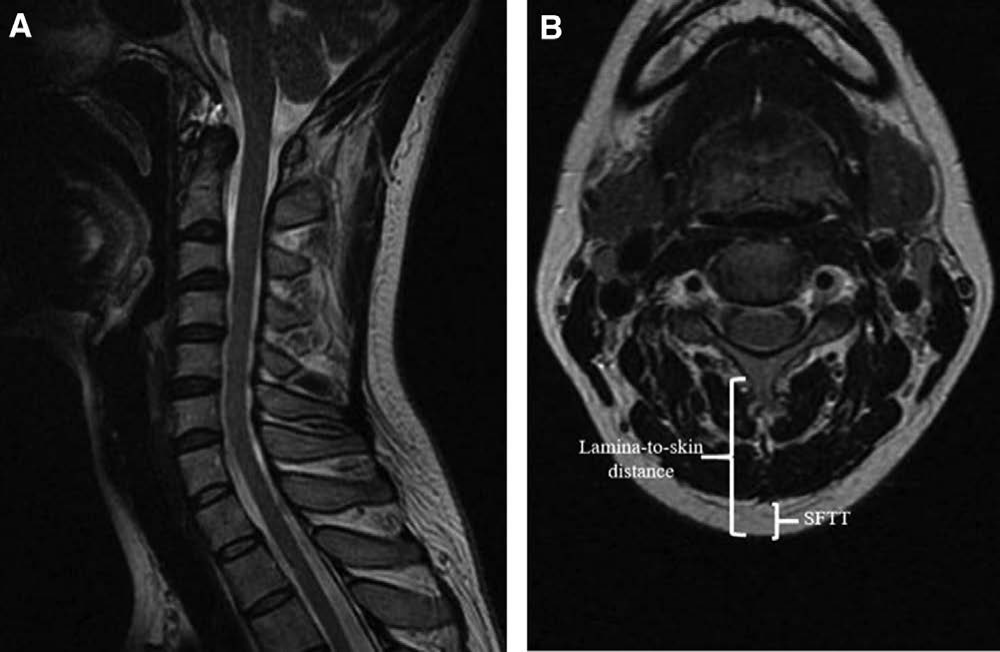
Measurement technique of SFTT at C3 level on T2-weighted axial cervical MRI. MRI = magnetic resonance imaging, SFTT = subcutaneous fat tissue thickness.
